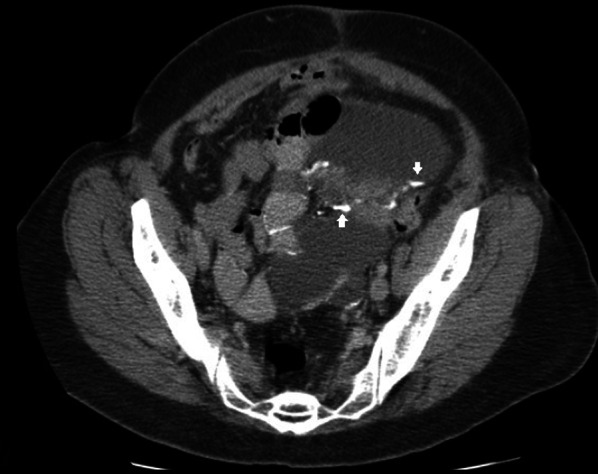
CT axial image of a 47-year-old male patient with cystic mesothelioma showing nodular calcifications along the cyst walls (arrows)
